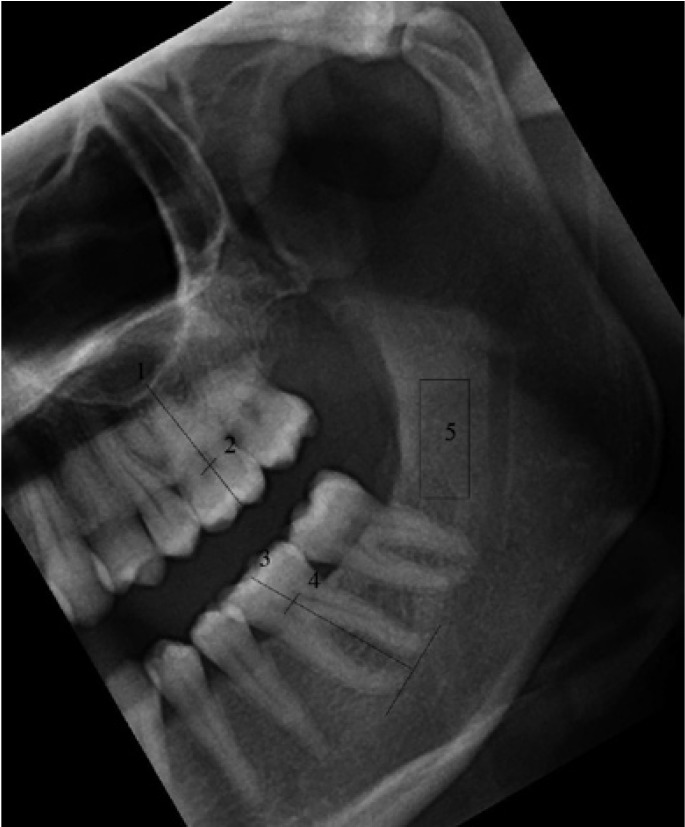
Cropped and rotated digital panoramic radiograph showing the measurement of maxillary (2) and mandibular (4) coronal pulp width, maxillary (1) and mandibular (3) tooth length and the region of interest placement (5) for fractal dimension calculations.
